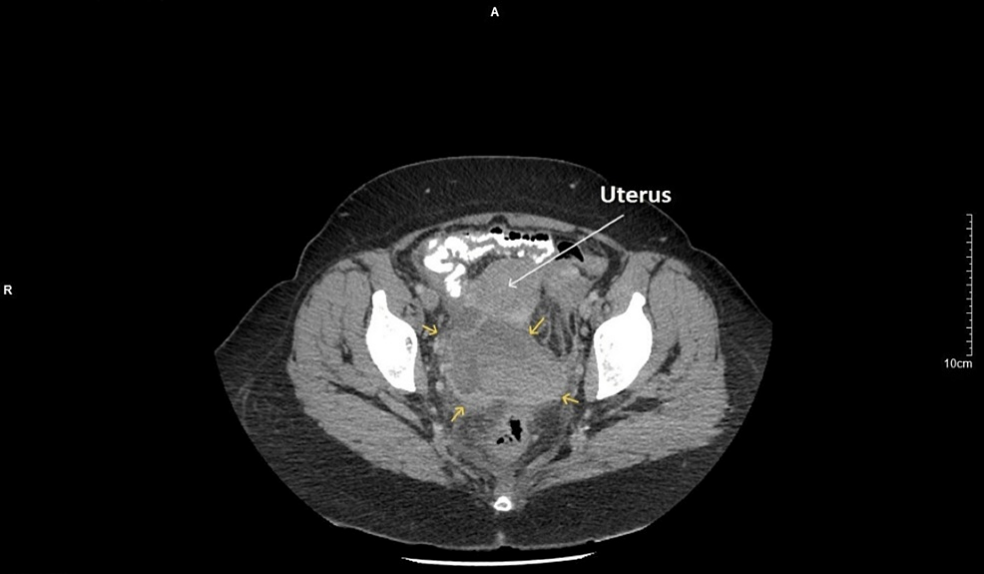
CT scan of a twisted mature cystic ovarian teratoma The posterior pouch of Douglas between the rectosigmoid and the uterus reveals the presence of a large mass (yellow arrows), which was misdiagnosed as an encapsulated pelvic inflammatory fluid collection that originated from the right adnexa.
